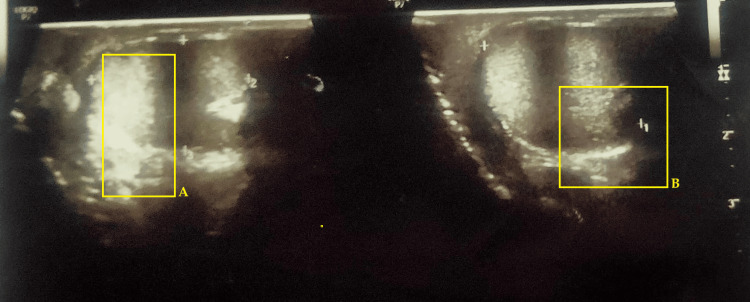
Ultrasound images of the hernial sac protruding through a defect in the anterior abdominal wall. A: right testis, B: left testis; testis not clearly visualized, tubular, mixed echogenic structures above/near testis noted as a classic for bowel in hernia sac.
